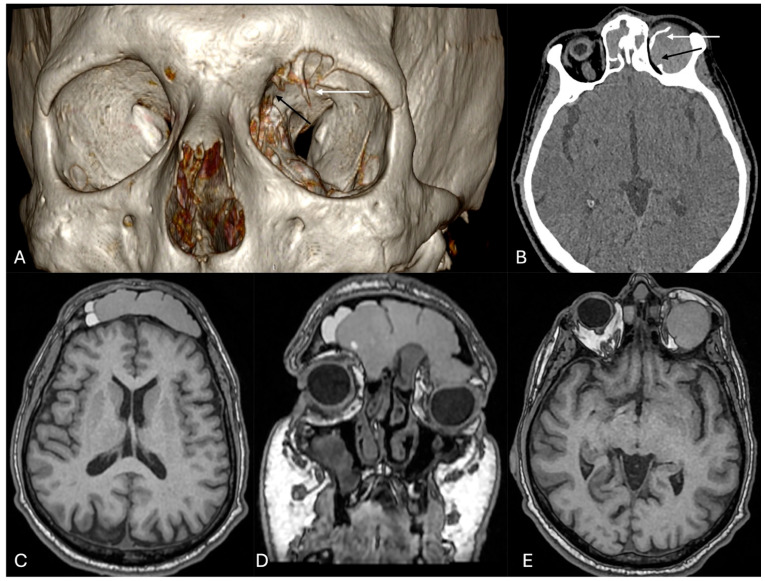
Preoperative diagnostic images. (A,B) Head CT scan: (A) 3D reconstruction and (B) axial sequence: a bony fragment protruding into the left orbital cavity (white arrows) and the bony erosion of the roof (black arrows) are evident; (C–E) contrast-enhanced brain MRI: axial (C), coronal (D), and axial (E) sequences: inhomogeneous contrast-enhanced lesion arising from the ethmoid sinus, occupying and occluding the frontal sinus (C,D), with extension into the left orbit (E).
